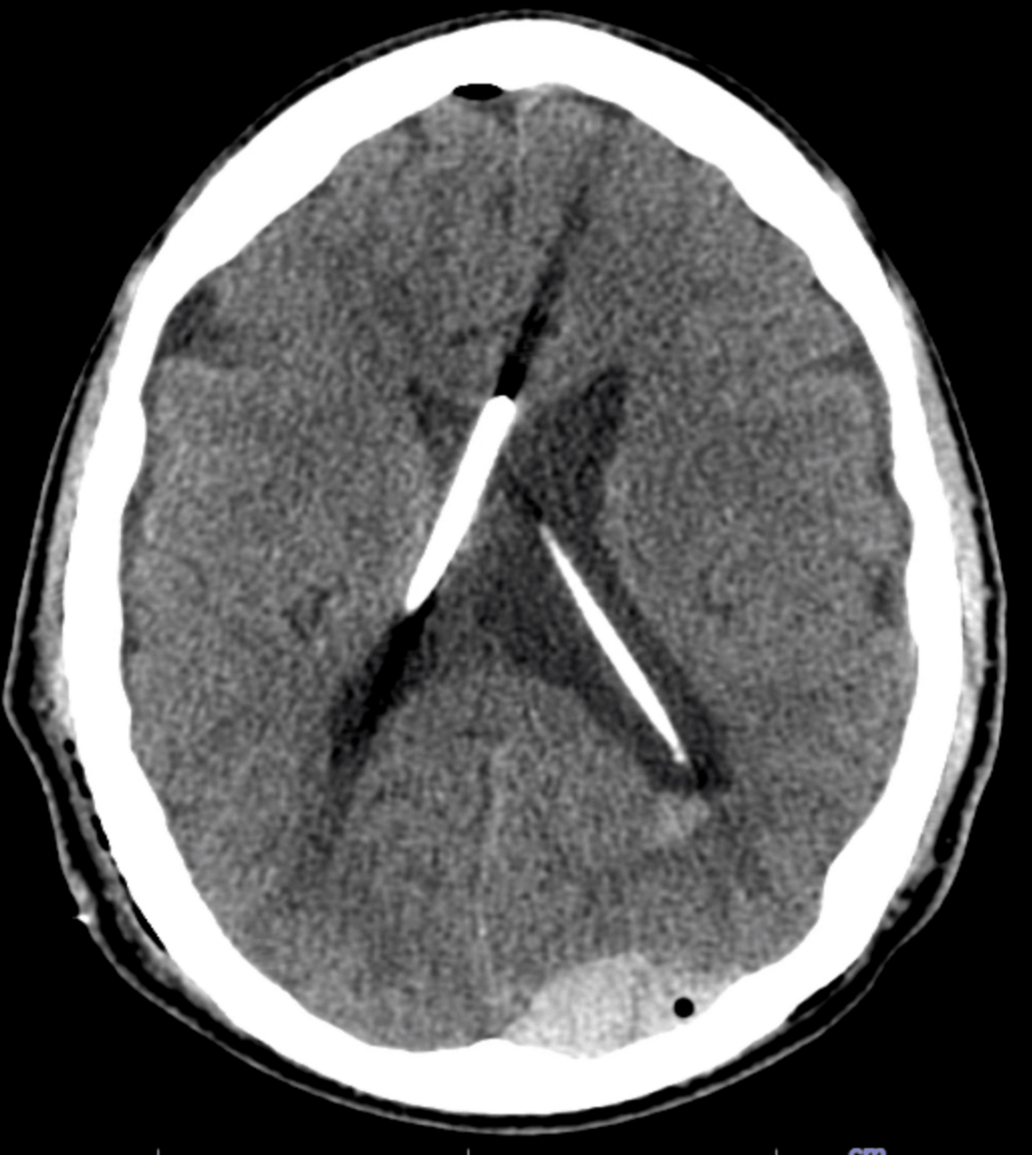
Axial CT obtained after left ventricular catheter placement, confirming bilateral catheter position and restoration of ventricular symmetry.
